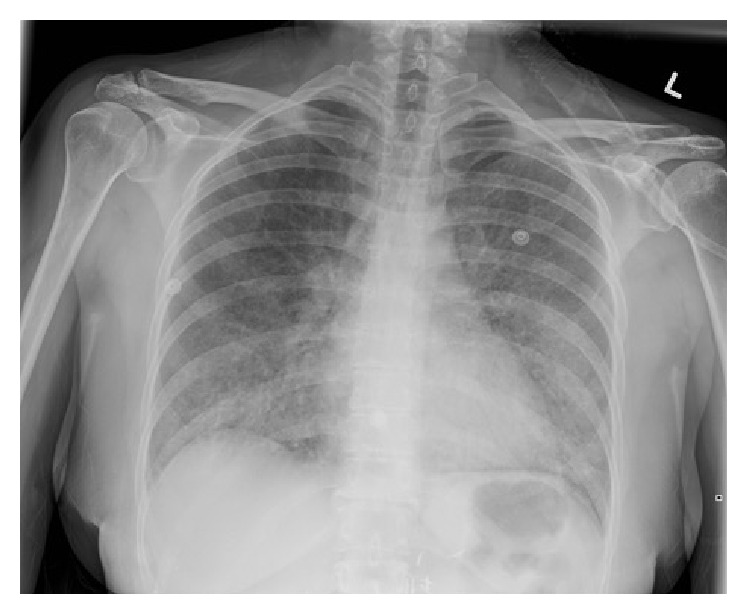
Initial chest radiograph, posterior-anterior view, showing hazy airspace opacification of the lower lobes bilaterally.
